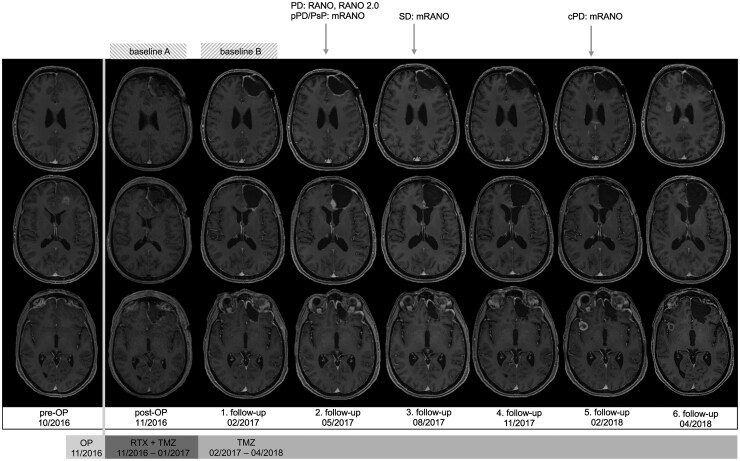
Response assessment on a representative patient. Patient (GB-0110): post-op MRI serves as baseline A for RANO, while the postradiation MRI/1.follow-up (21-35 days after the last day of radiotherapy) serves as baseline B for mRANO and RANO 2.0. At the second follow-up MRI, tumor progression is suspected because a new measurable lesion appeared (<1 cm^3^), and PD is defined according to RANO. Since this scan was obtained more than 12 weeks after completion of radiotherapy, PD is also defined according to RANO 2.0. In contrast, mRANO classifies this finding as preliminary PD. At the third follow-up MRI, a reduction in tumor volume is observed; therefore, SD is defined according to mRANO, and the prior MRI (second follow-up) is retrospectively classified as pseudoprogression. Tumor volume remains stable through the fourth follow-up. However, at the fifth follow-up MRI, a new lesion is detected, leading to a diagnosis of PD according to mRANO. Abbreviations: cPD, confirmed progressive disease; mRANO, modified response assessment in neuro-oncology; PD, progressive disease, pPD, preliminary progressive disease, PsP, pseudoprogression; RANO, response assessment in neuro-oncology; RTX, radiotherapy; SD, stable disease; TMZ, temozolomide.
